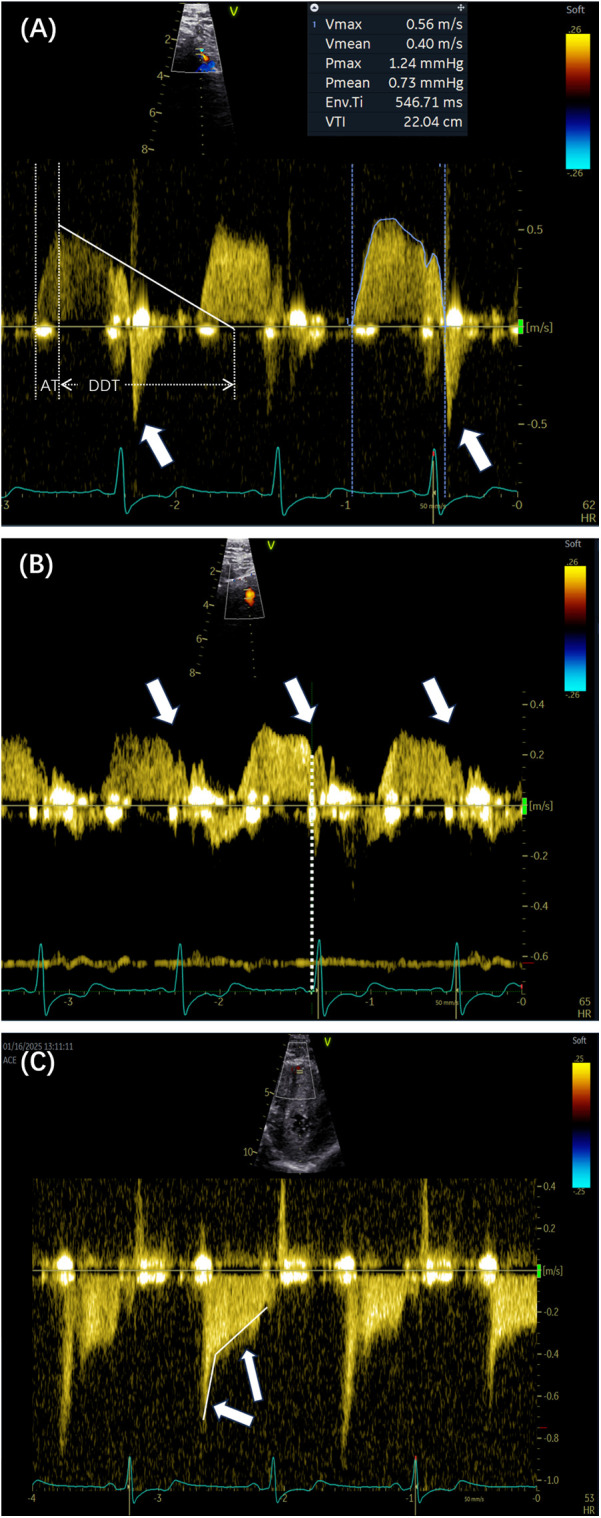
(A) The outline of the Doppler spectrum was traced to calculate various parameters, including the peak velocity, mean velocity, and velocity–time integral. The white lines indicate the DDT and AT. The arrows show the presence of SFR (arrows). (B,C) Two Doppler spectrum features are indicated by the sudden dip in end-diastolic velocity (arrows) and the corresponding time point of the dip (dashed lines). The square root sign patterns are indicated by arrows. DDT, diastolic deceleration time; AT, acceleration time; SFR, systolic flow reversal.
